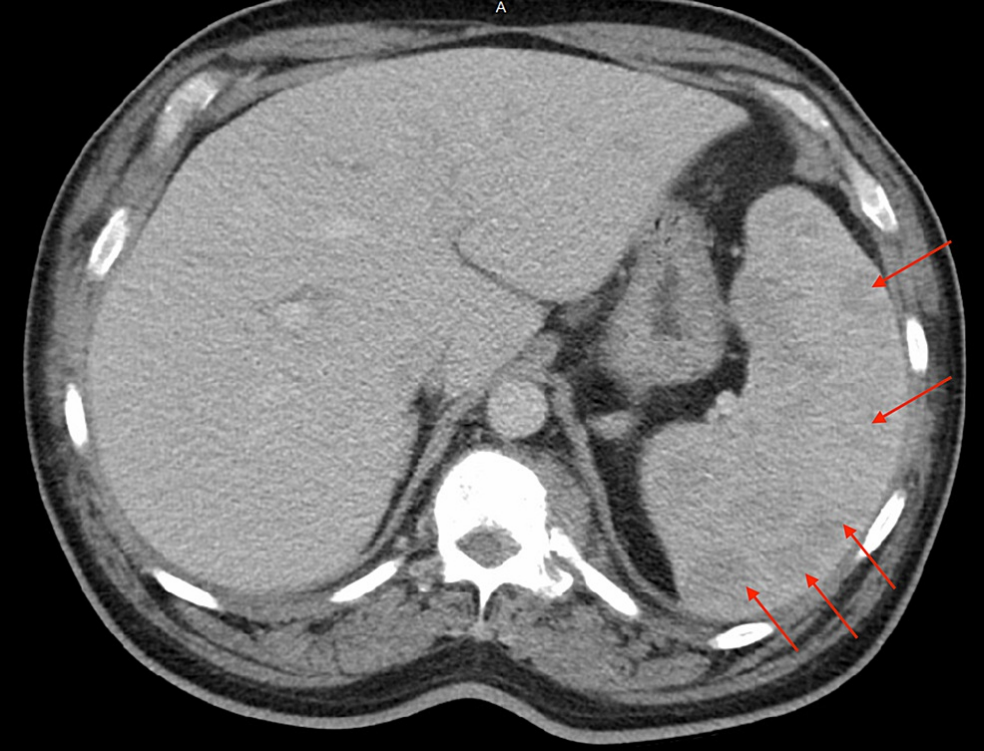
CT abdomen/pelvis showing splenomegaly with multiple hypodense lesions
